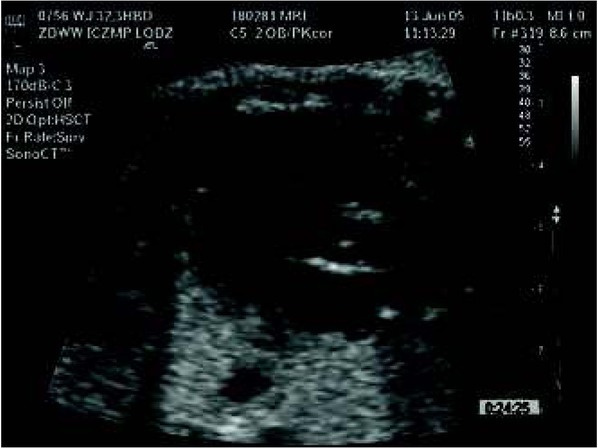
One of the fetal echocardiography scans of the left ventricle in semi long view with chordea tendinea (arrow), which later on might be a cause of the heart murmur during auscultation, despite normal heart anatomy. Ryc. 4. Jeden z przekrojów serca płodu w czasie badania echokardiograficznego ukazujący w świetle lewej komory serca nitkę ścięgnistą (strzałka), która może być przyczyną szmeru u dziecka z prawidłową budową serca.
